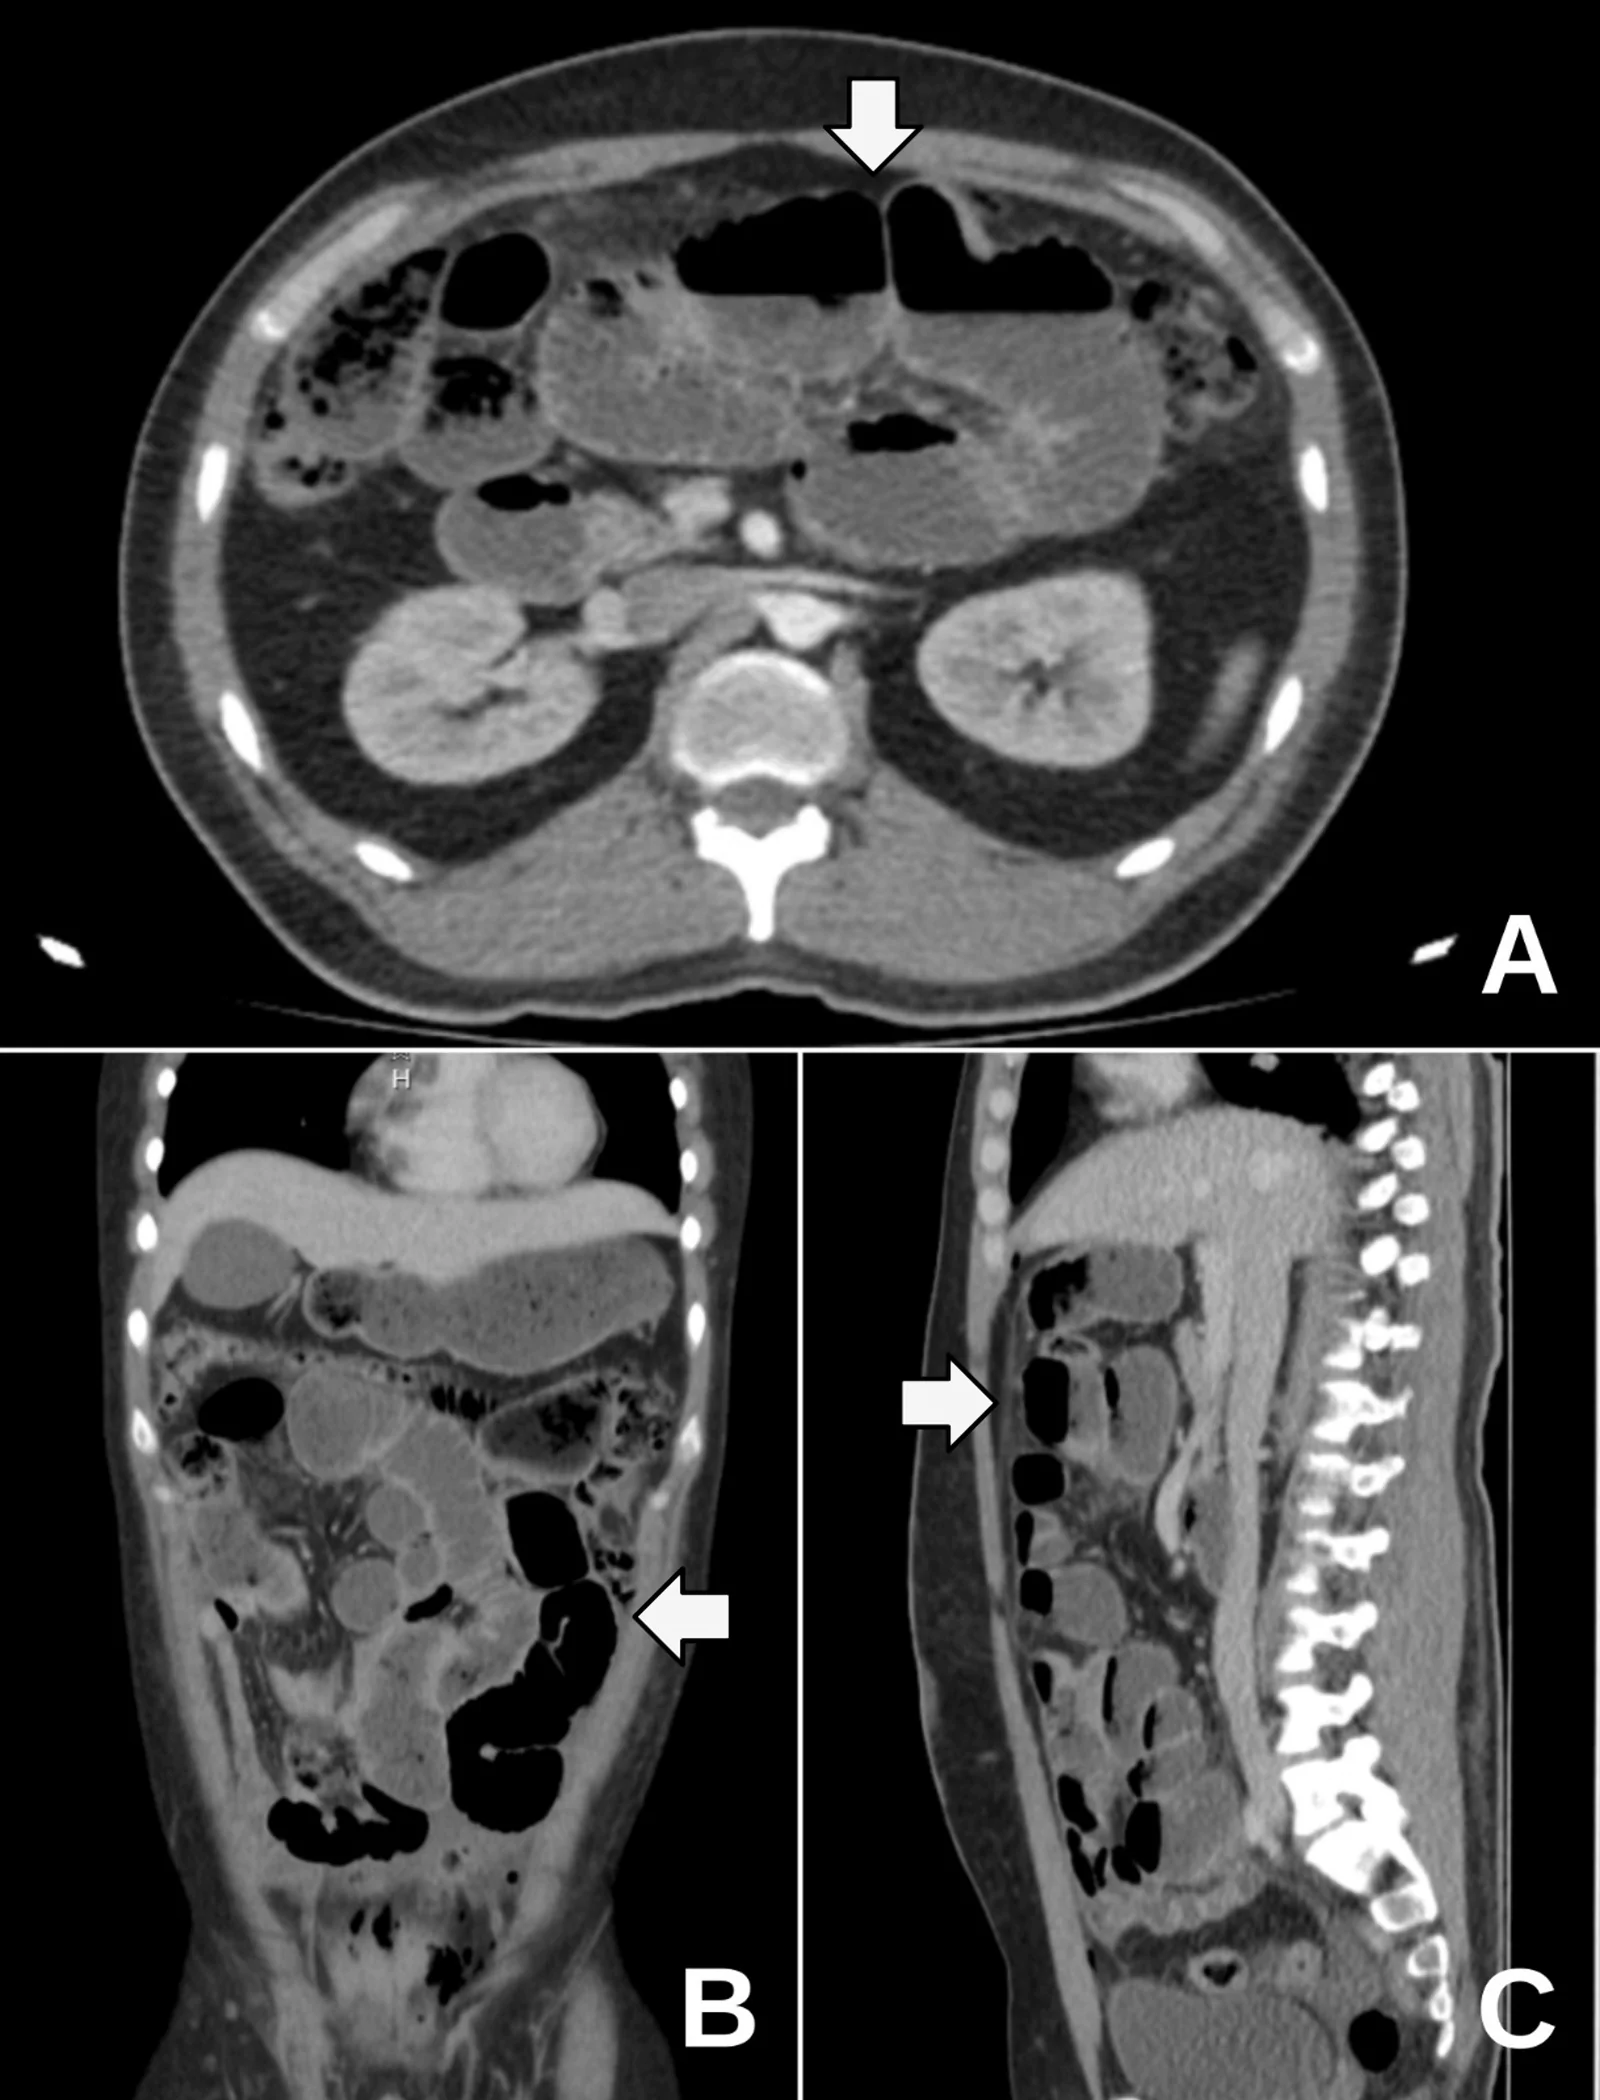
Follow-Up Contrast-Enhanced Thoracoabdominal CT Demonstrating Postoperative Paralytic Ileus Contrast-enhanced computed tomography (CT) in axial section (A) and coronal (B) and sagittal (C) reconstructions demonstrating diffuse dilation of small bowel loops with the predominance of fluid content and multiple air-fluid levels, without the identification of a definite transition point. These findings are consistent with paralytic ileus
